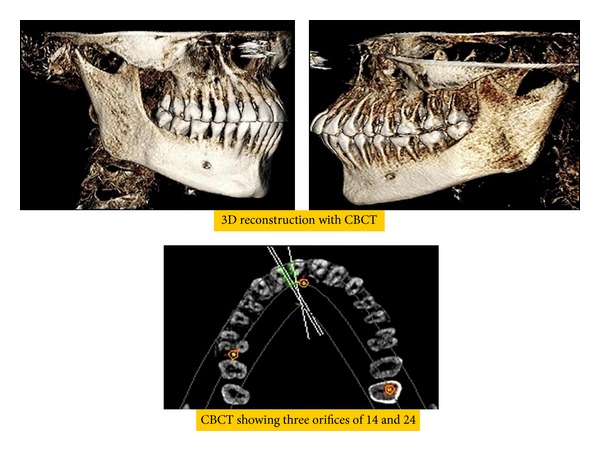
CBCT images of 14 and 24.
